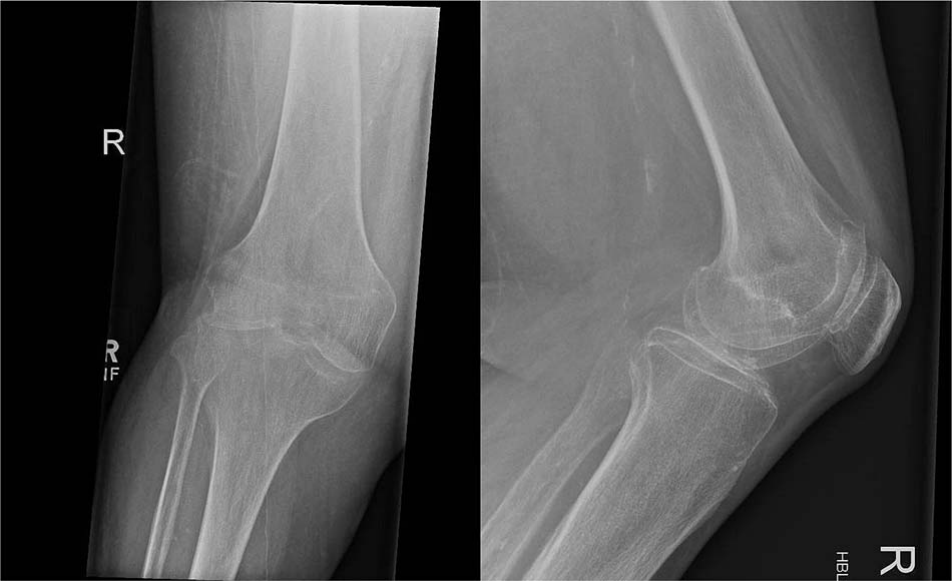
Pre-operative AP and lateral X-rays of the knee.
